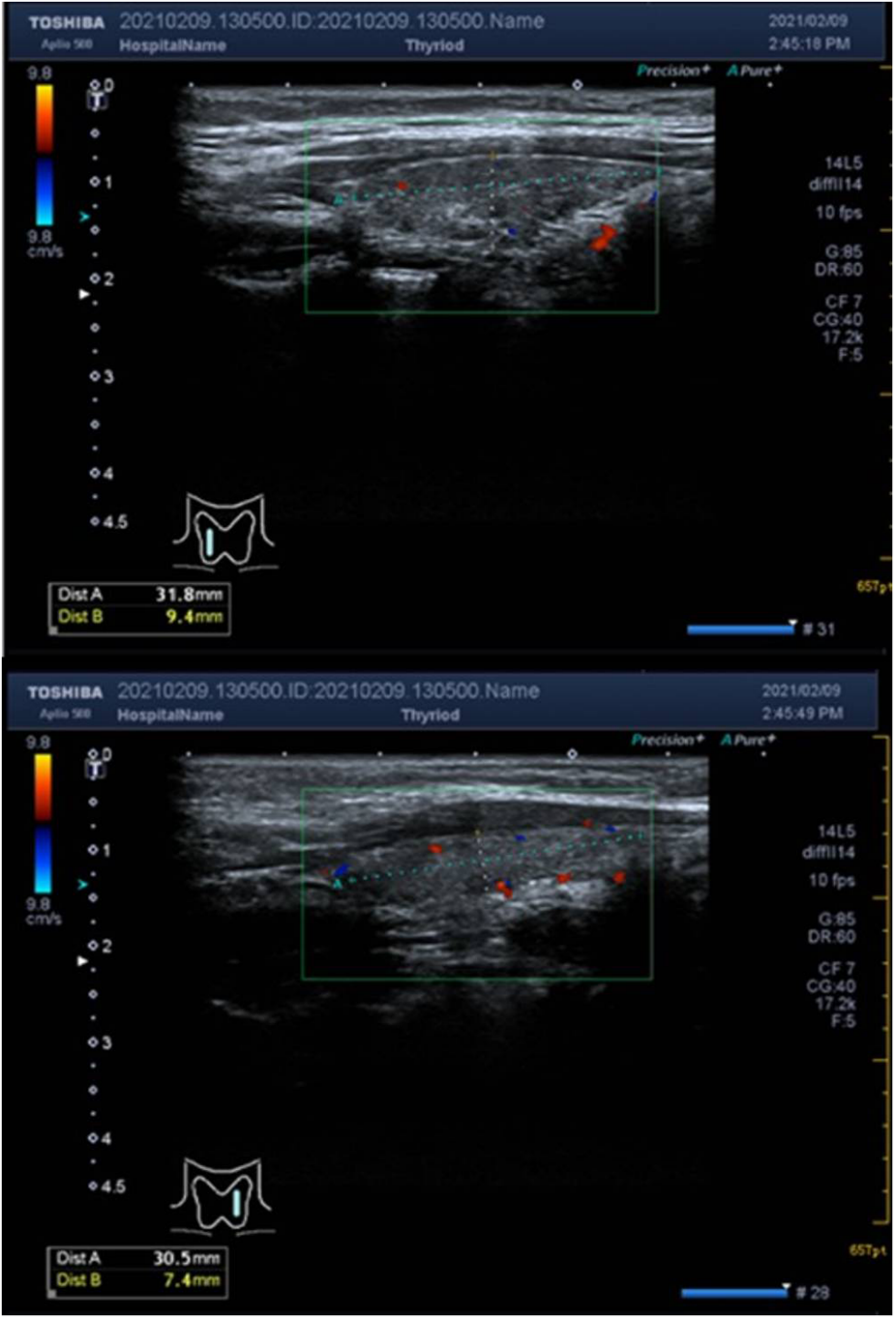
The dashed line above the thyroid ultrasound shows the size of the anterior-posterior and superior-inferior diameters. The anterior-posterior diameter of the right lobe of the thyroid gland was 0.9 cm, the left and right diameters were 1.1 cm, the upper and lower diameters were 3.2 cm, and the anterior-posterior diameter of the left lobe was 0.7 cm, the left and right diameters were 1.0 cm, the upper and lower diameters were 3.1 cm, and the thickness of the isthmus was about 0.14 cm. The parenchymal echogenicity was hypoechoic and inhomogeneous, no exact occupancy was seen, and no significant abnormal blood flow signal was detected.
